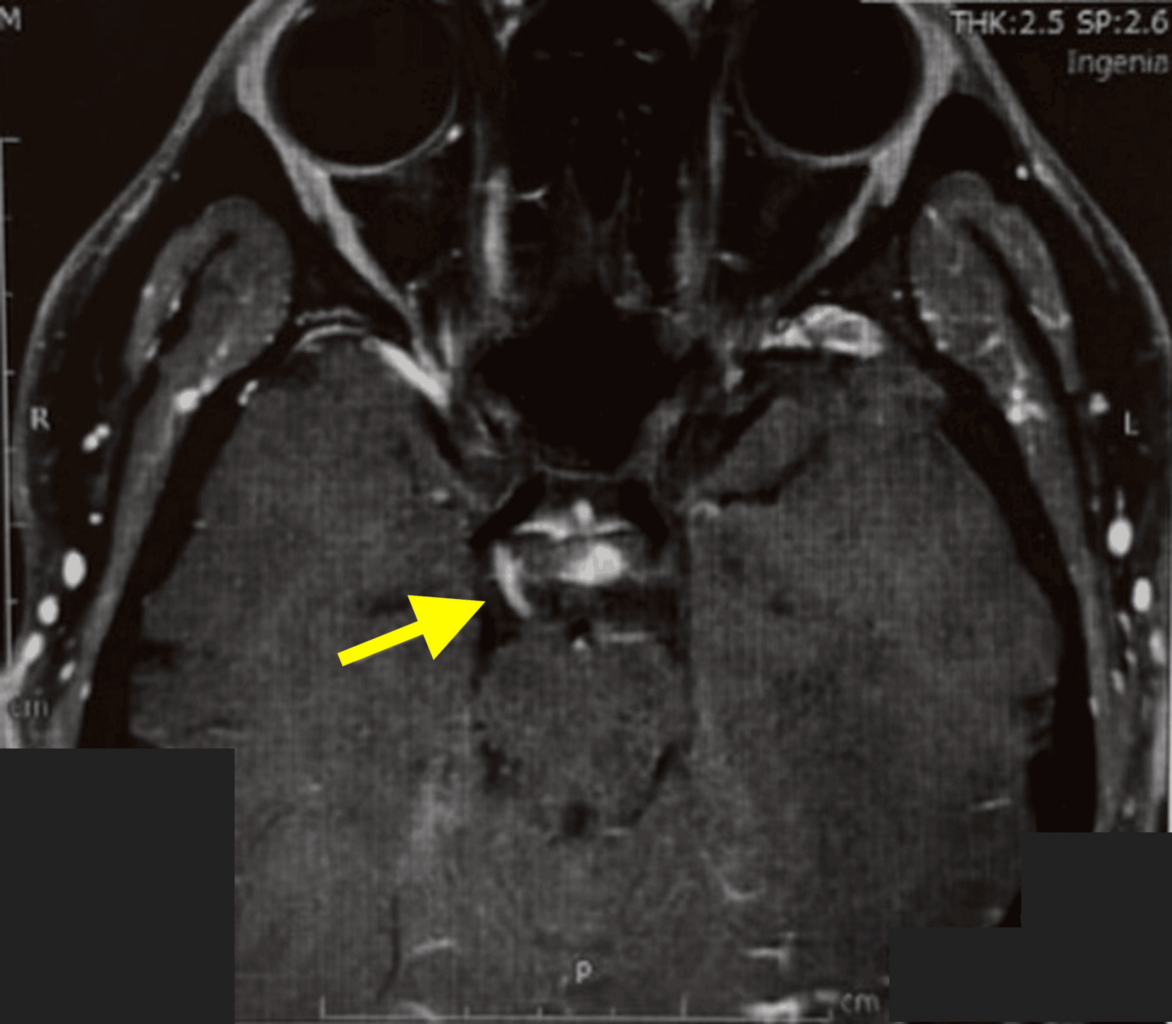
Contrast-enhanced brain MRI Brain MRI demonstrated swelling and enhancement of the right oculomotor nerve (yellow arrow).
